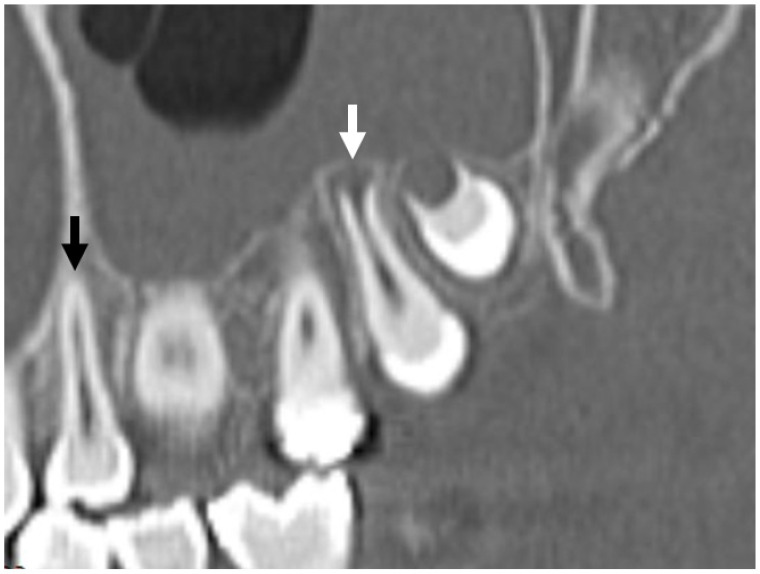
Obliquely reformatted CT demonstrating a mature root apex of the maxillary first premolar (black arrow) and the developing root of maxillary second molar palatal root. Note the flared appearance of the root apex with a “blunderbuss” appearance (white arrow). The increased lucency around the apex should not be mistaken for pathology.
